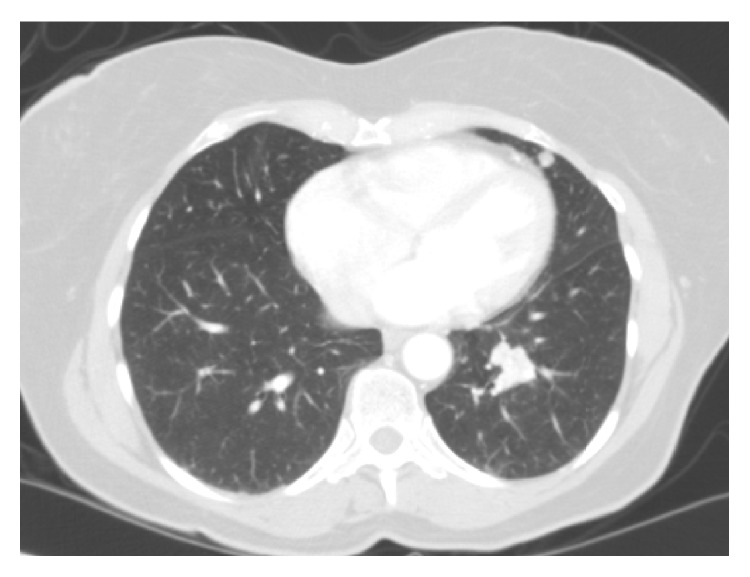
Imaging study. Computed tomography of the chest reveals a 2.0 × 1.6 cm mass and a stable nodule in the left lower lobe.
